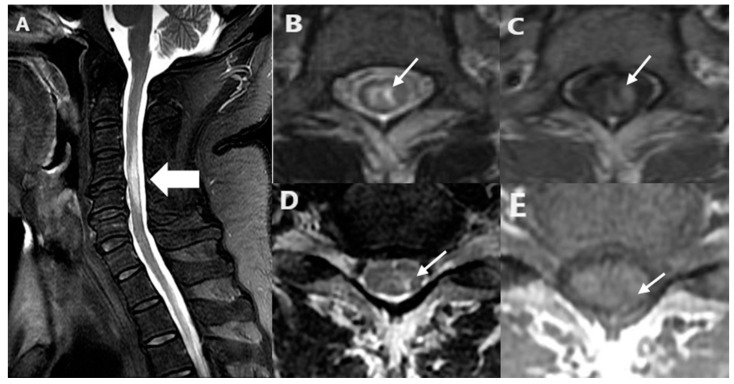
Spine MRI images of NTNC myelitis patients. (A) Sagittal T2-weighted images of cervical myelitis patient with sagittal BSLs (thick white arrow). Thoracic myelitis patient with centrally located BSLs (B,C) and cervical myelitis patients with peripherally located BSLs (D,E) on axial views. BSLs show very hyperintense lesions as high as the surrounding CSF on the T2-weighted image (thin white arrow) (B,D) and partially hypointense not as lowas the surrounding CSF on the T1-weighted image (thin white arrow) (C,E), which is different from permanent tissue loss.
